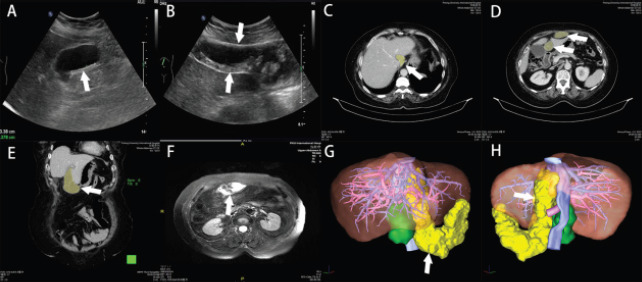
A panel of US, CT, MR, and Three-Dimensional reconstruction. (A) US showing sludge and sand particles in the gallbladder (arrow). (B) US showing a solid cystic mass on the abdominal wall (between the two arrows). (C) Axial CT view of the caudate lobe of the liver (arrow and yellow area). (D) Axial CT view of liver fissure zone and abdominal wall (arrows and yellow areas). (E) Coronal CT view of abscess of LTH (arrow and yellow area). (F) MR of a cystic mass with solid mass (arrow). (G) 3D reconstruction of the shape of LTH (arrow and yellow area). (H) 3D reconstruction of the ligament Arantius (arrow and yellow area)
